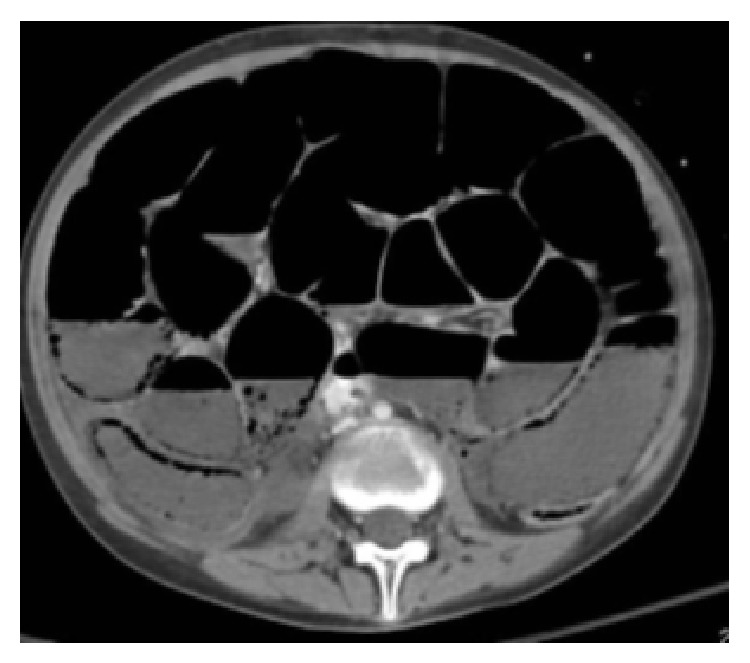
Computed tomography showing air in the intestinal wall.
